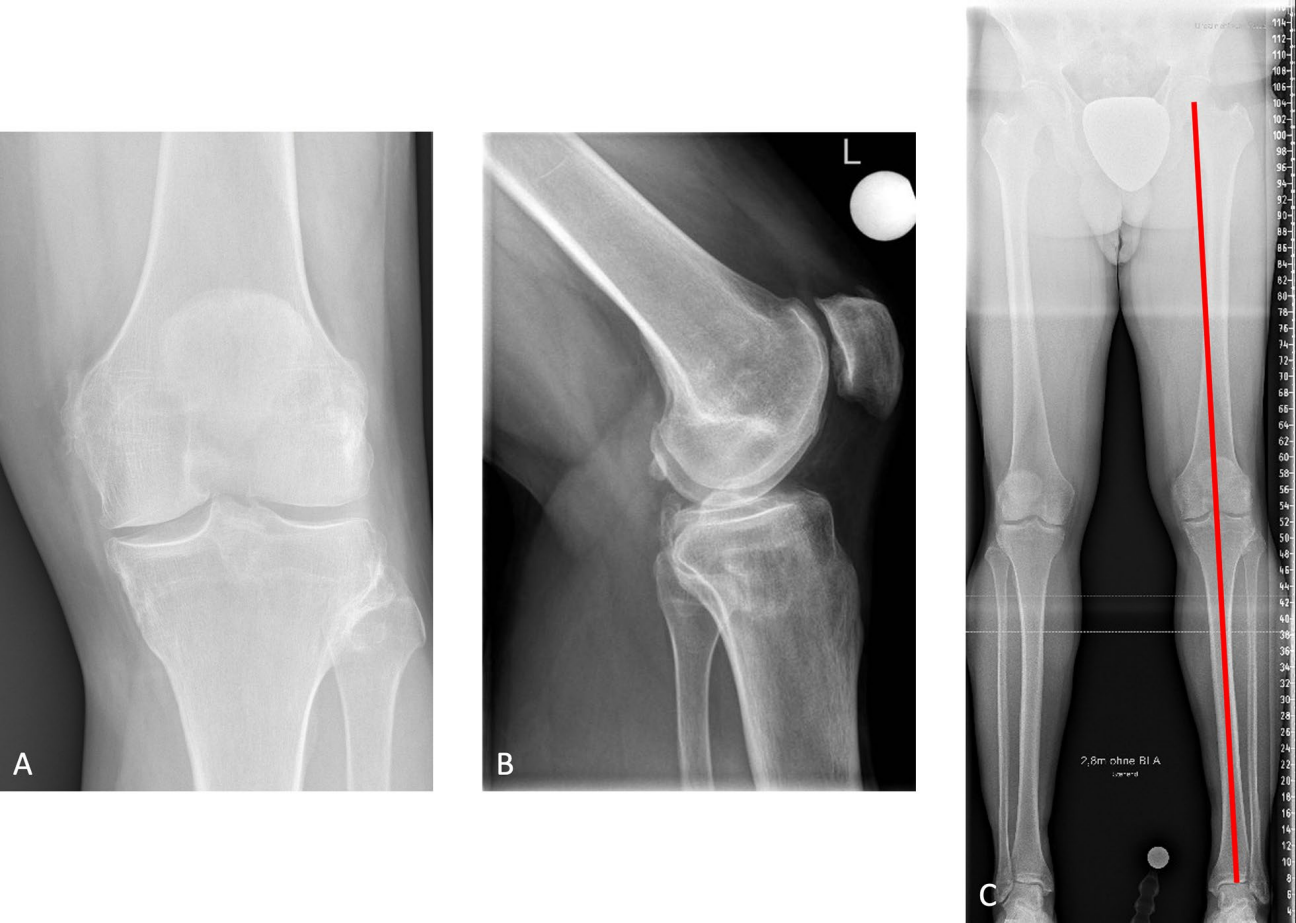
Anteroposterior (A) and lateral (B) radiographs of 52-year-old male, 37 years after Phemister epiphysiodesis of the left distal femur and proximal tibia, showing osteoarthritis of the knee joint Kellgren and Lawrence grade I but regular intra-articular joint anatomy and physiological limb alignment (C)
